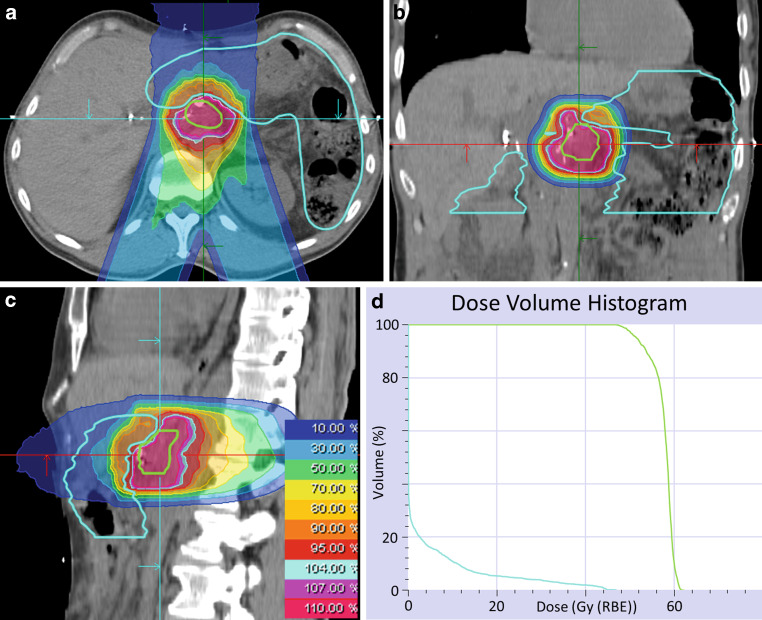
Radiation plan of a 59-year-old patient suffering from locally recurrent pancreatic cancer demonstrating a steep dose gradient of the performed irradiation. The patient was irradiated in supine position using two oblique posterior beams to avoid gastrointestinal toxicity. Axial (a), coronal (b), and sagittal (c) computed tomography (CT) slices and isodose lines are shown. The gross tumor volume (GTV) is delineated in *green*, the gastrointestinal tract is contoured in *light blue*. Isodoses represent a forward-calculation using an α/β ratio of 2 Gy in the local effect model (LEM) I. Therefore, the GTV seems to be overdosed. In the actually irradiated plan, an α/β ratio of 5 Gy in LEM I was used for the tumor tissue. d Dose–volume histogram of the radiation plan demonstrating adequate coverage of the GTV (*green*) while avoiding overdosage in the gastrointestinal tract (*light blue*)
